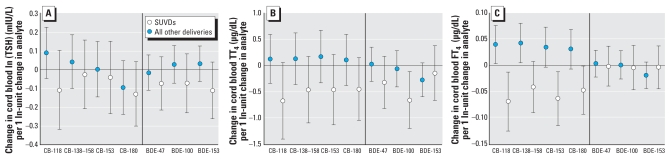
Change in cord blood thyroid hormones associated with a 1 ln-unit change in analyte by birth delivery mode. (*A*) Cord blood TSH (natural log adjusted). In addition to the covariates above, this model also adjusted for history of STDs and parity. (*B*) Cord blood TT_4_. In additional to the covariates above, this model also adjusted for history of STDs. (*C*) Cord blood FT_4_. In addition to the covariates above, this model also adjusted for reported hypertension, diabetes, and anemia. SUVDs, *n =* 92 with available data for the multivariate models. All other deliveries, *n =* 194. All models adjusted for baby’s sex, gestational age, maternal age, maternal race, maternal prepregnancy BMI, and smoking status. Error bars represent 95% CIs.
